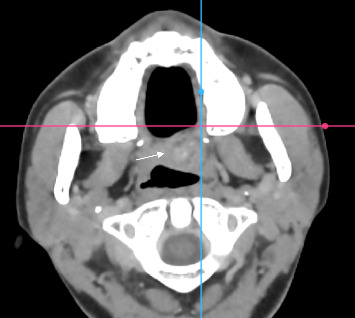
A computed tomography scan of the well-defined soft-tissue mass measuring 2.5 × 2.0 cm, clearly separated from the surrounding structures.
